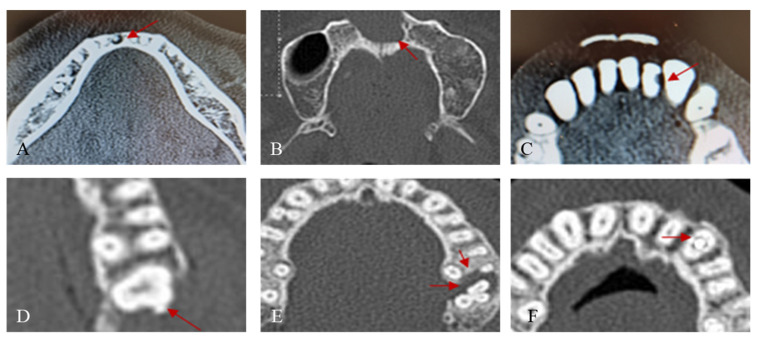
Representative oral lesion criteria in CT image. (A) Periapical abscess. (B) Cystic lesion. (C) Dental caries. (D) Calculus. (E) Periodontitis (F) Tooth fracture.
